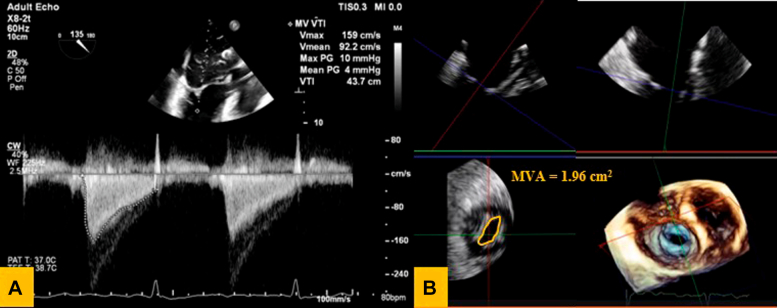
Postinterventional Transesophageal Echocardiography, Patient 1 (A) A drop in the mean transmitral gradient to 4 mm Hg. (B) An increase in mitral valve area (MVA) to 1.96 cm^2^ measured by 3-dimensional planimetry.
